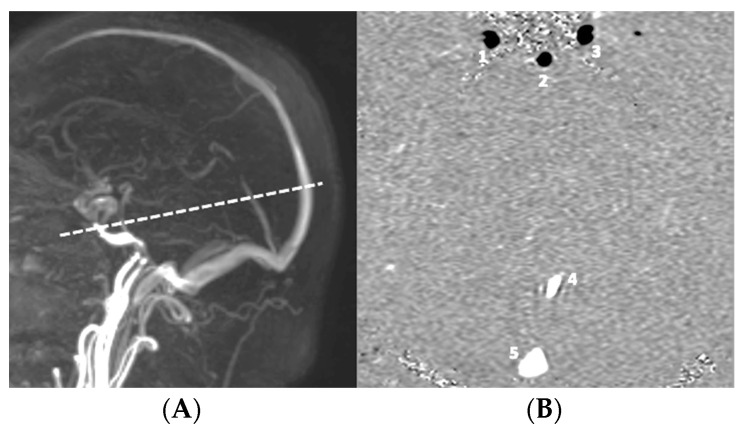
Slice plane position for vascular acquisition of phase-contrast MRI for hemodynamic evaluation. (A) Position of a 3D time-of-flight sequence; (B) axial slice of phase-contrast MRI (encoding velocity = 60 cm/s) through 1—right carotid artery; 2—basilar artery; 3—left carotid artery; 4—straight sinus; 5—superior sagittal sinus.
